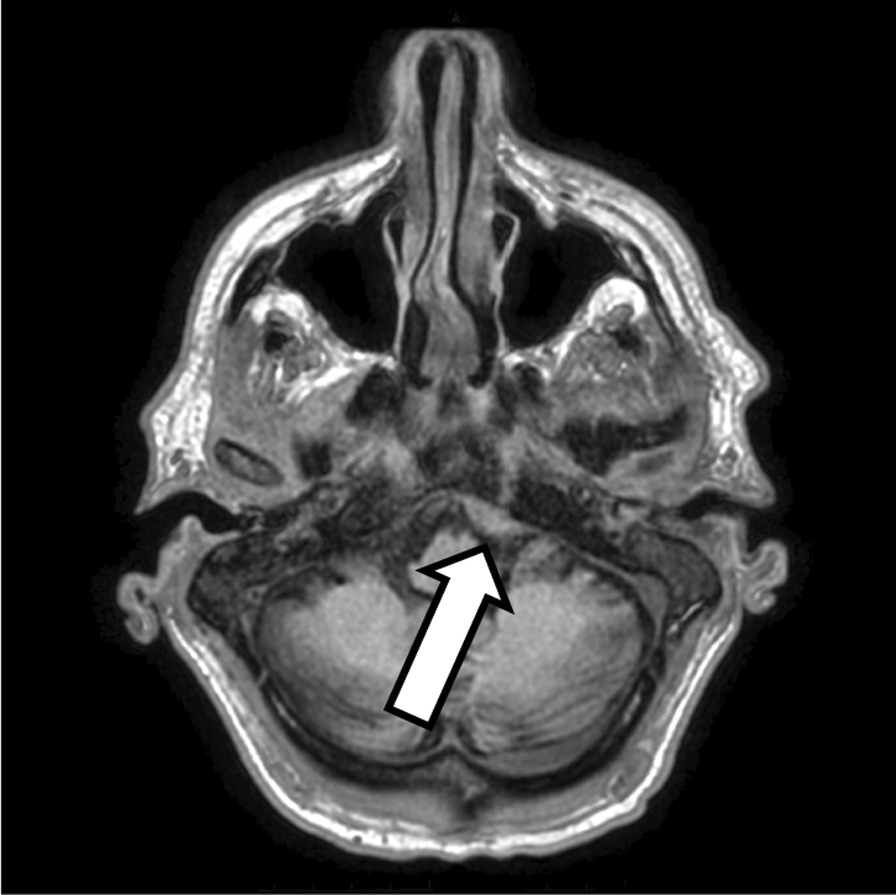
T1-weighted brain MRI demonstrating a thickening of the meninges that was initially considered a meningioma but subsequently re-diagnosed as a gumma (left, arrow)
